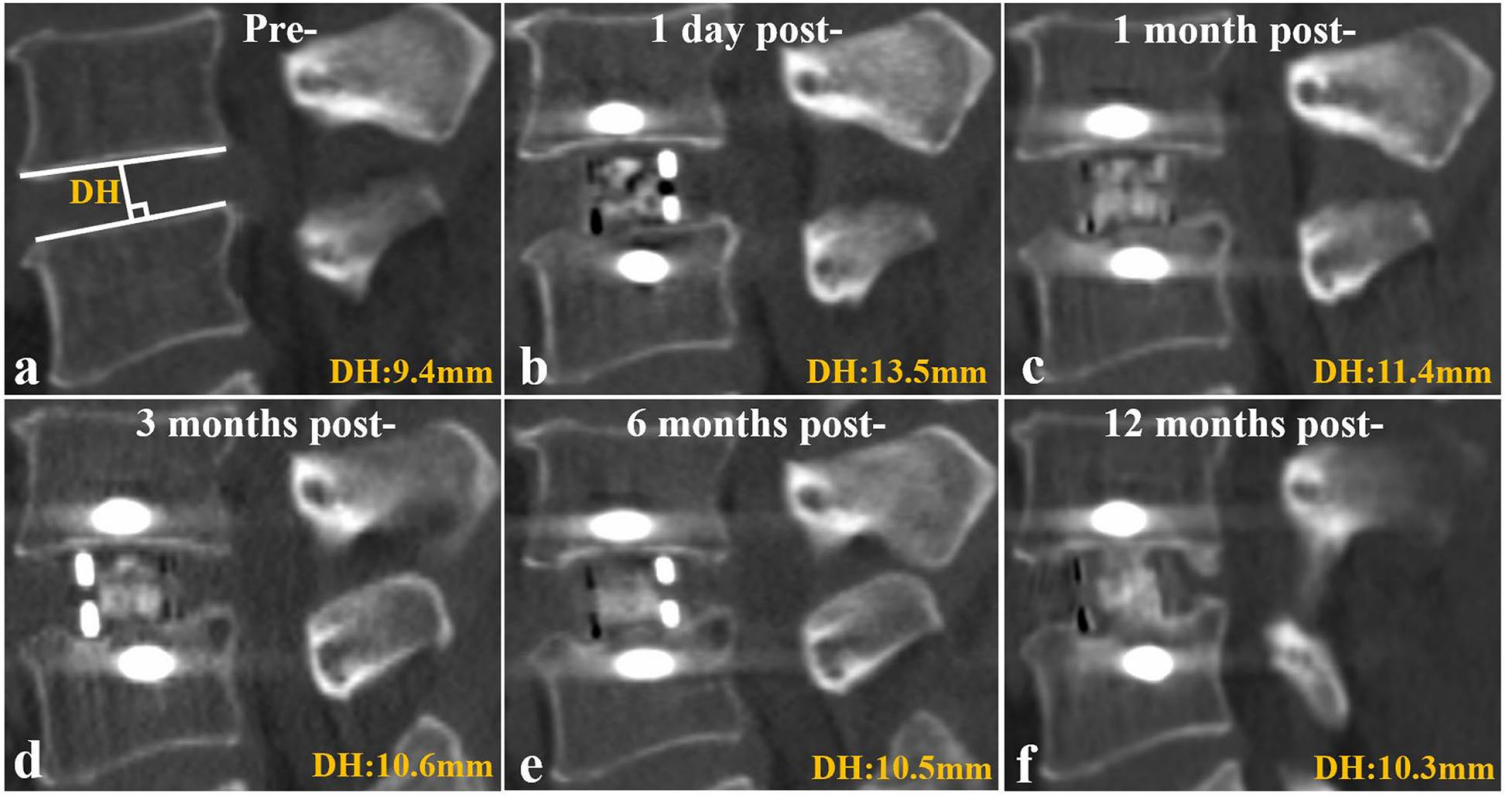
Cage subsidence (CS) course of a 63 years old man. Disk height (DH) was measured as the vertical distance between the midpoint of the cranial endplate and the caudal endplate on the CT midsagittal plane (a). CS was identified at 1 month post- (c), and obviously progressed at 3 months post- (d), while did not significantly change at 6 (e) and 12 (f) months post-. Pre-, preoperative; post-, postoperative
